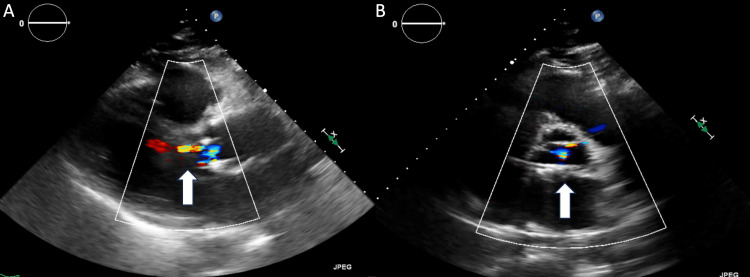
Transthoracic echocardiogram on postoperative day eight (A, B) Left ventricular ejection fraction > 55%, mild aortic insufficiency (white arrow)
